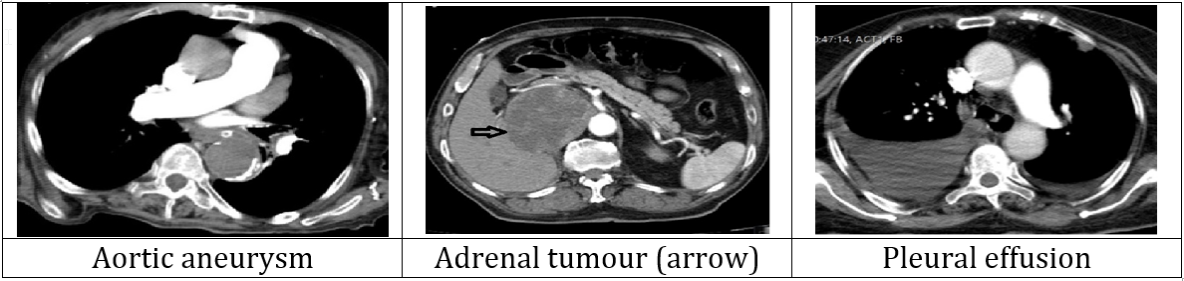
Examples of incidental finding among cases that underwent computed tomography angiography
